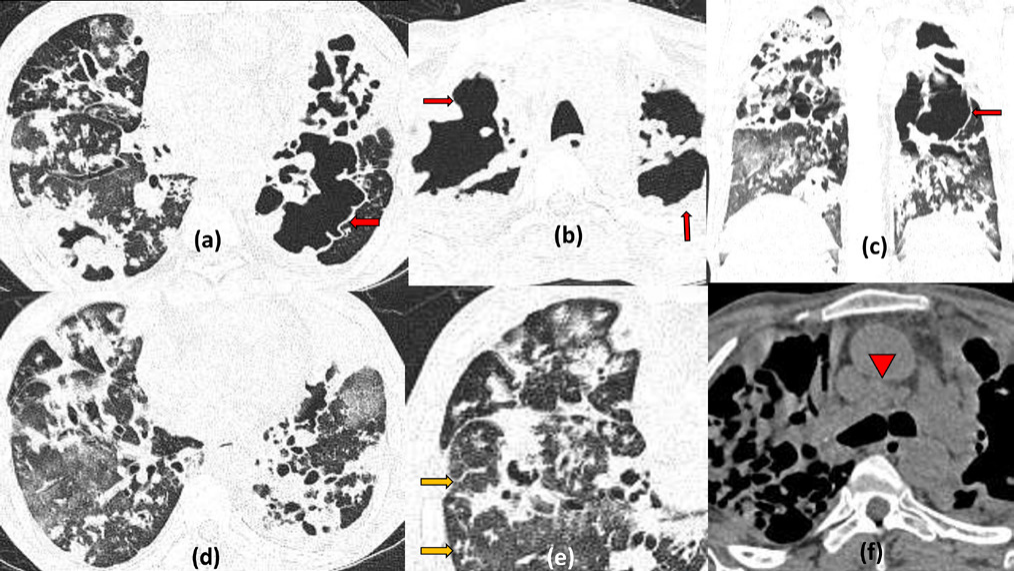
Reactivation *Mycobacterium tuberculosis* coinfection with COVID19 forming multiple cavities: 38-years-old COVID positive male with past history of pulmonary tuberculosis, presented to the emergency with respiratory distress. Chest HRCT axial and coronal lung window sections (a–e) shows multiple variable sized cavities with irregular walls predominantly in upper lobes (red arrows), multifocal patchy consolidation and centrilobular nodules (few showing tree in bud appearance distributed in bilateral lung fields (yellow arrow in zoomed image e). Lower lung fields also show patchy ground glass opacities suggestive of acute COVID19 infection. Axial mediastinal window (non-enhanced - f) shows few subcentimetric pre-carinal lymph nodes (arrowhead). Positive results were obtained of sputum CBNAAT for *Mycobacterial tuberculosis* and RTPCR for SARS-CoV-2 virus confirming coinfection.
